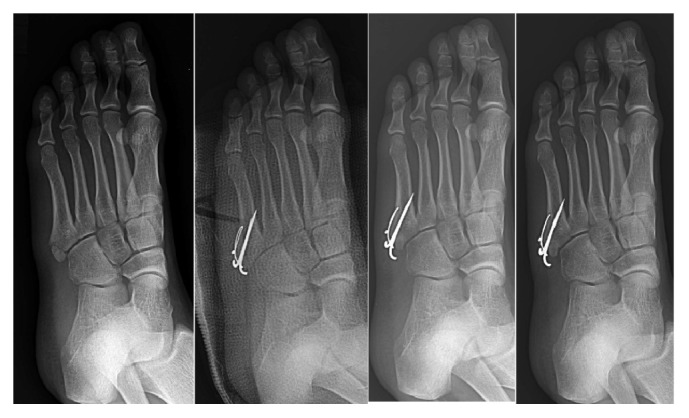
Operative treated, early weight-bearing patient's radiograph. 24-year-old female; radiograph of preoperation and 1-, 3-, and 6-month follow-up (from left to right).
